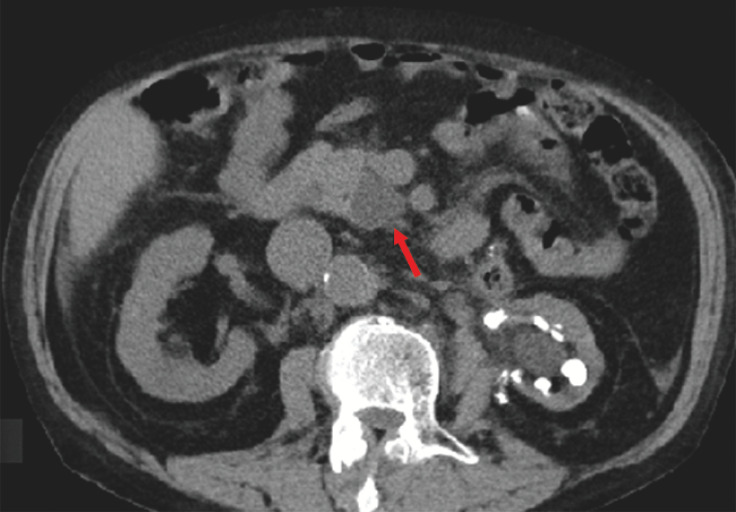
Computed tomography shows a cystic lesion in the pancreatic head (red arrow).
